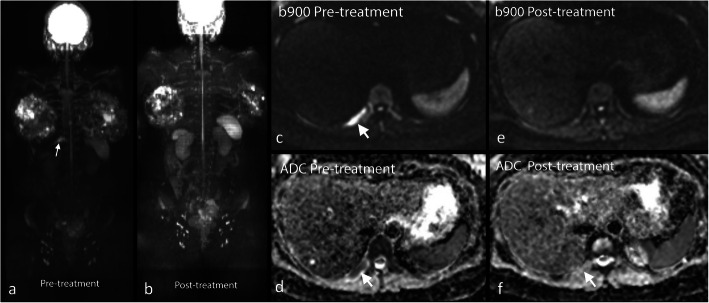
WBDWI in bone disease following chemotherapy. WB-MRI in a 59-year-old woman with breast cancer. 900 MIP before (a) and after (b) chemotherapy as well as b-900 and ADC maps pre (c and d) and post (e and f) chemotherapy demonstrate a right 12th rib lesion on with high DWI signal and low ADC on the pretreatment study (white arrow d) and complete response of the lesion after treatment (e and f)
